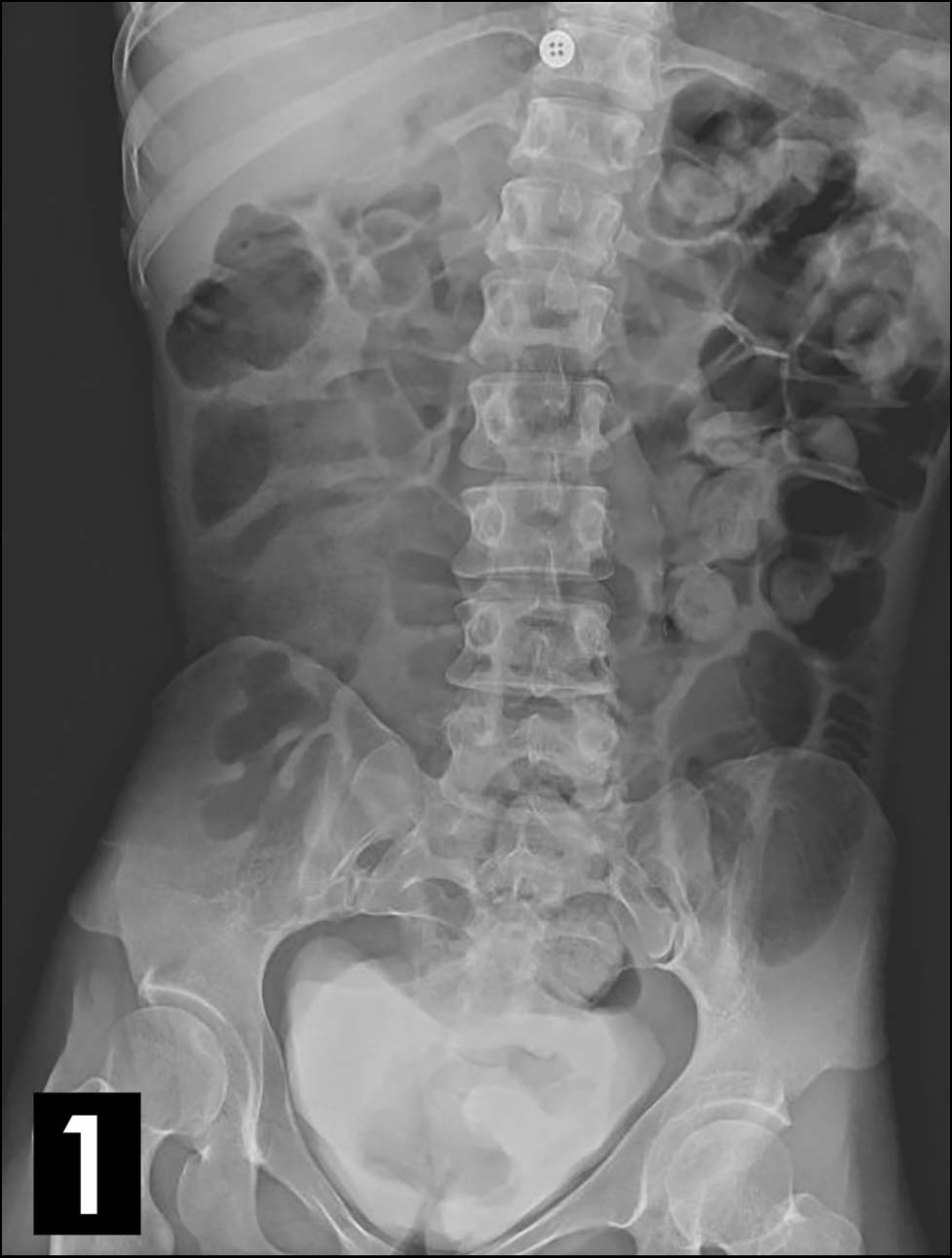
Abdominal X-ray demonstrated mildly dilated small bowel loops with gas throughout the large bowel.
